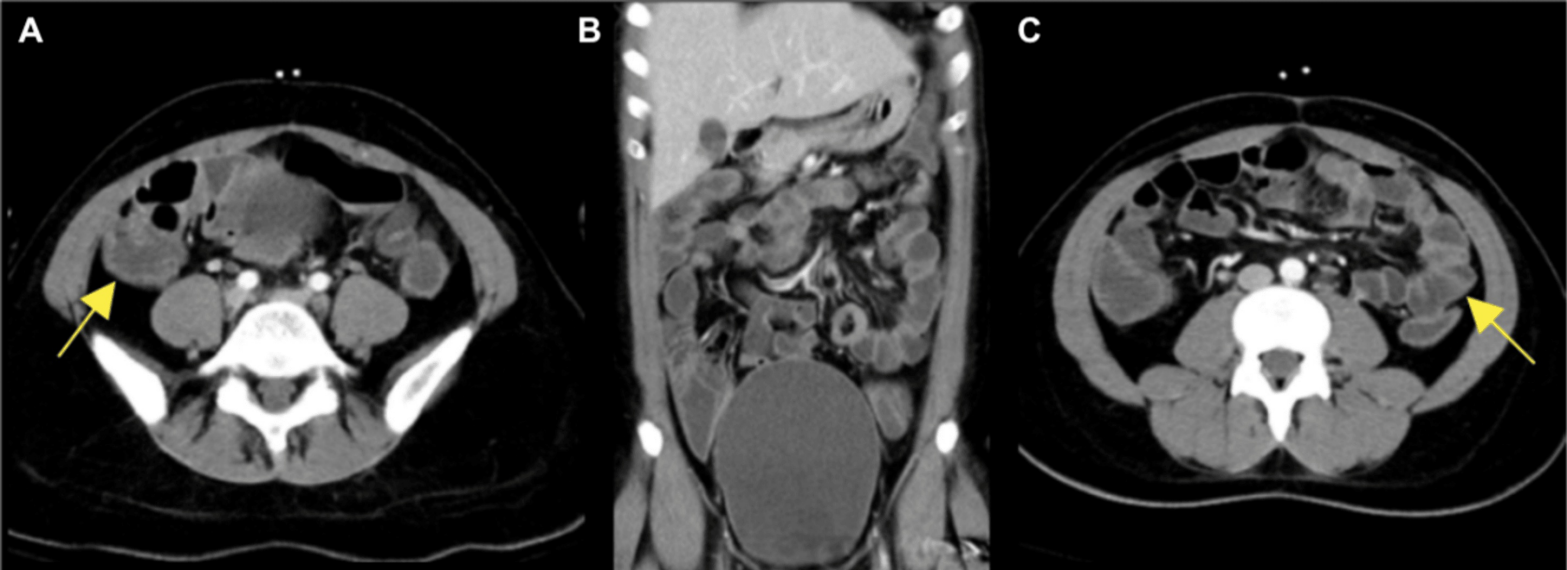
Axial (A, C) and coronal (B) CT images of the abdomen and pelvis with intravenous contrast demonstrating diffuse bowel wall thickening (yellow arrows) suggestive of enterocolitis.
